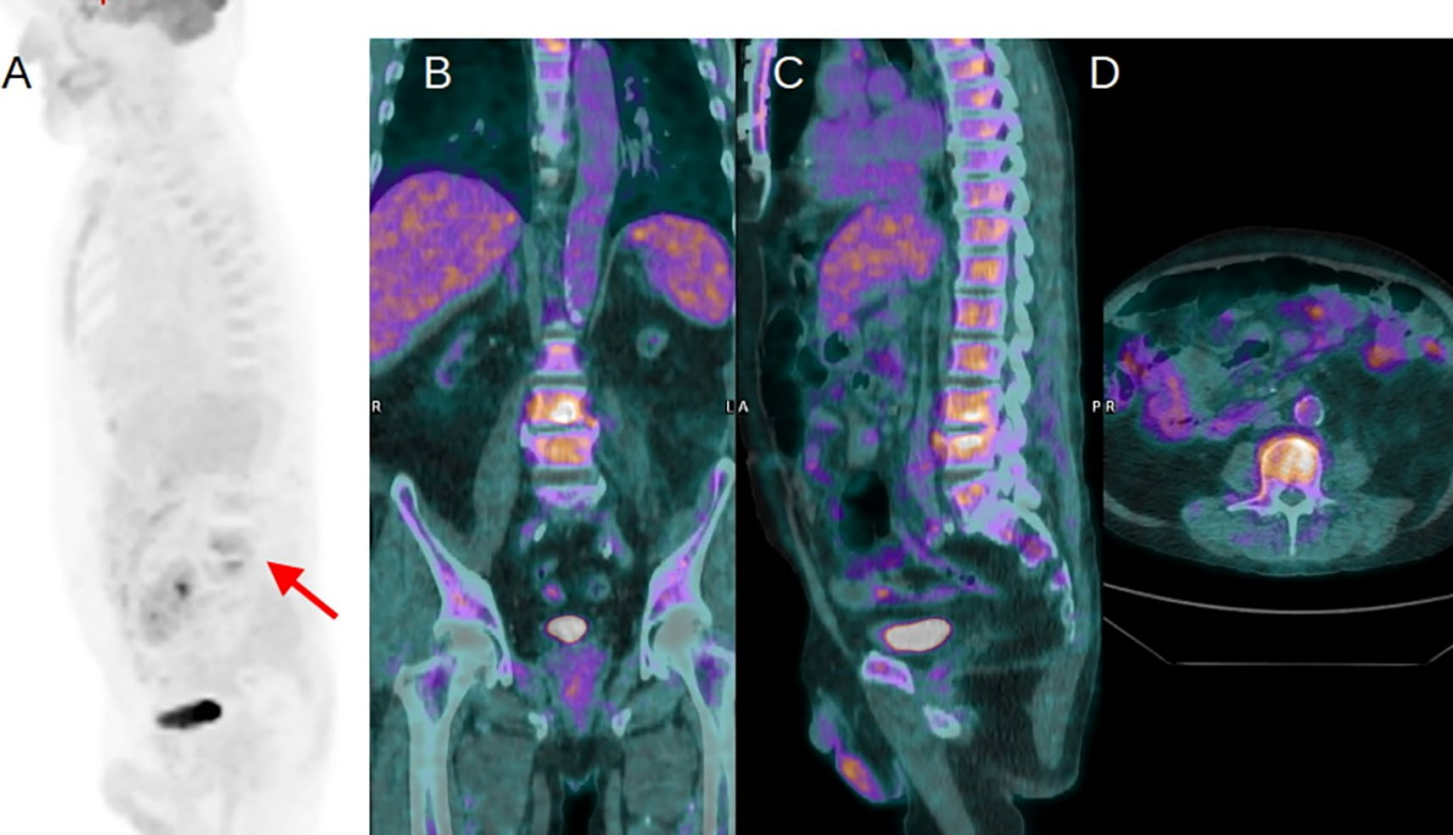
A 69-year-old man was admitted to the ICU with fever and fatigue after a prior abdominal surgical procedure. Abdominal ultrasound and thorax-abdominal CT were negative. Blood cultures were positive for *Staphylococcus aureus.* Maximum intensity projection PET (A) revealed the presence of an increased uptake in the column corresponding to the third and fourth lumbar vertebra. Subsequent coronal (B), sagittal (C) and axial (D) PET/CT fused images confirmed the increased 2-[^18^F]FDG uptake in the intervertebral disk and adjacent vertebra suspected for spondylodiscitis
